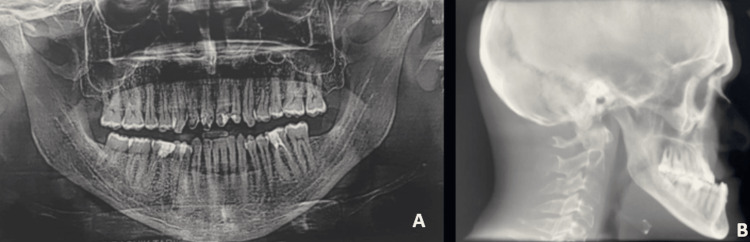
Preoperative panoramic radiograph and lateral cephalometric radiograph (A) Panoramic view. (B) Lateral cephalogram
